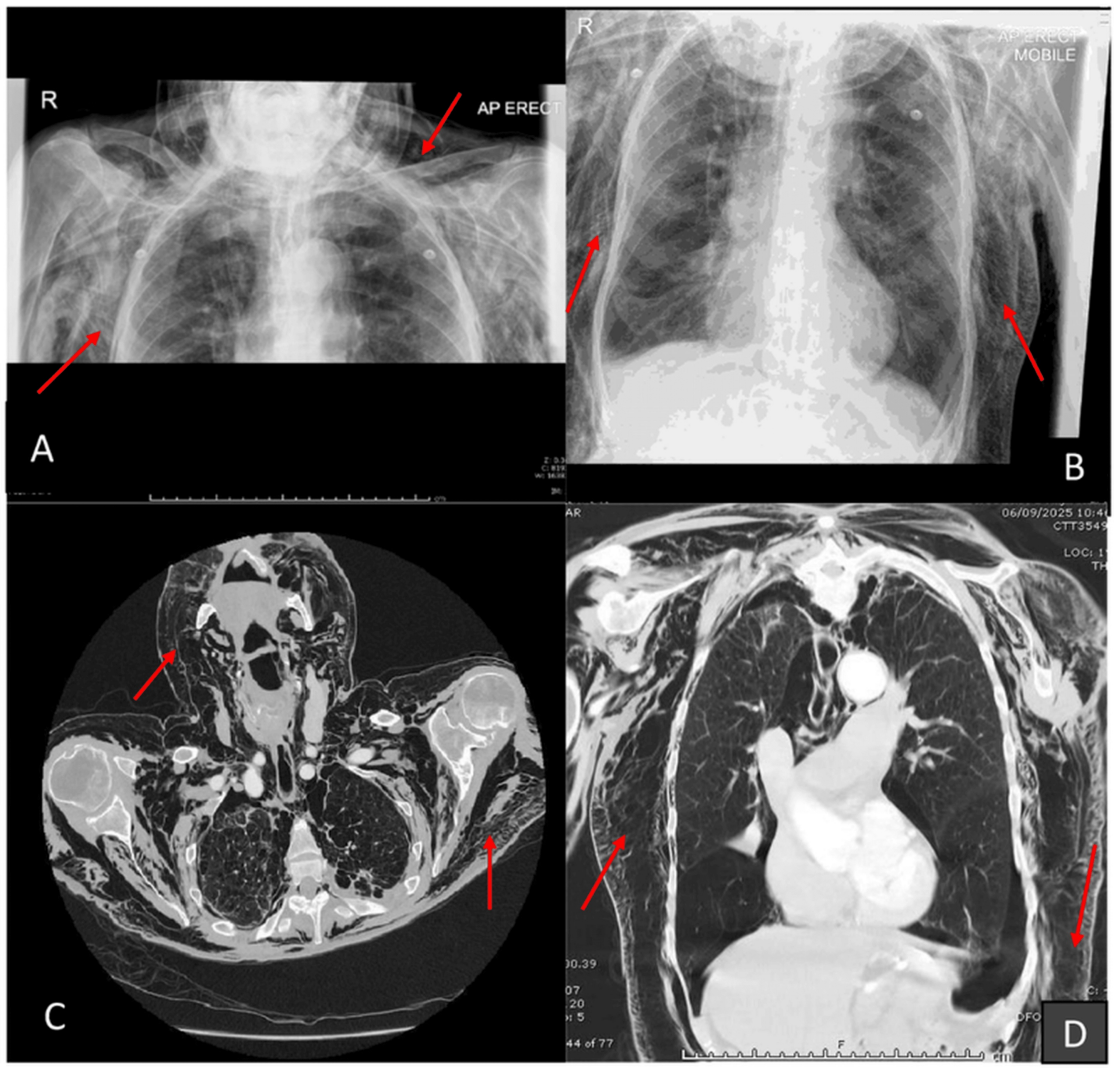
(A, B) Chest radiographs demonstrating extensive surgical emphysema extending into the neck and abdomen (red arrows). (C) Axial CT image showing marked subcutaneous emphysema extending from the chest wall into the neck. (D) Coronal CT image depicting extensive emphysema extending inferiorly into the abdominal region. Red arrows indicate areas of subcutaneous emphysema. Orientation markers and standard lung-window settings were used.
